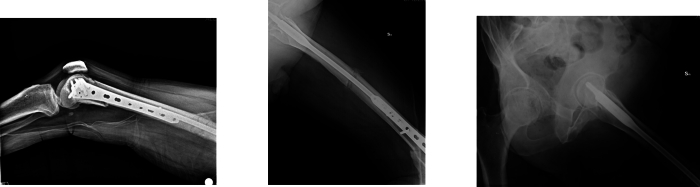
Control X-ray one month after surgery.
